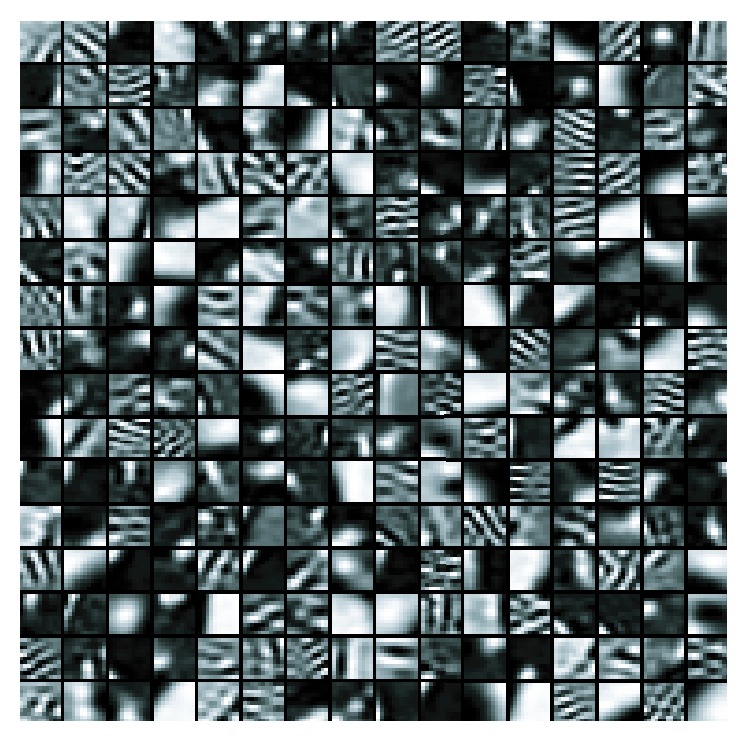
The codebook learned for CT scans on ART phase. The patch size is 11 × 11, and codebook size is 256.
